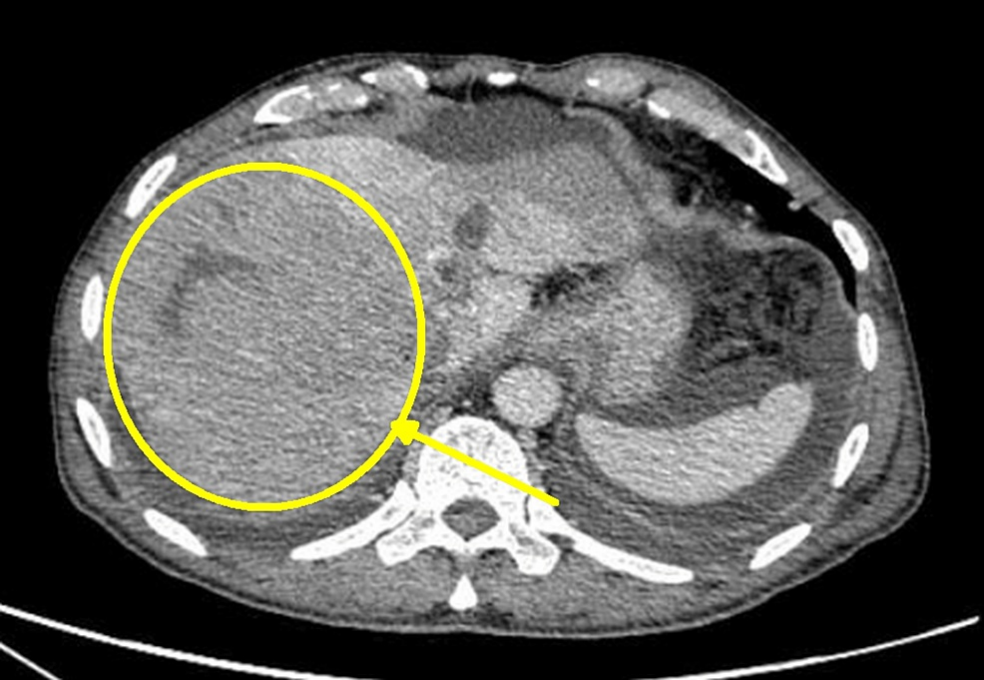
Dynamic hepatic CT scan revealing a large hypodense lesion in the right lobe of the liver causing compression of the right hepatic vein (arrow) CT: computed tomography
